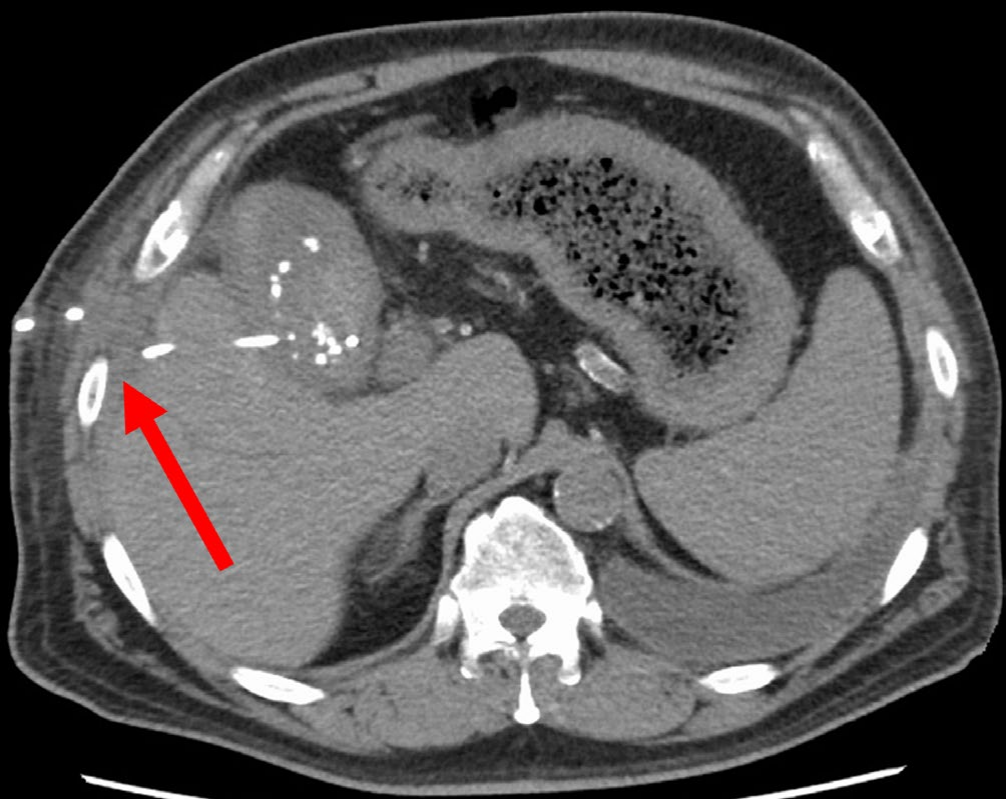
Case 1. Abdominal computed tomography image taken after percutaneous transhepatic gallbladder drainage placement at the previous hospital. The catheter is placed through the eighth intercostal space (arrow).
